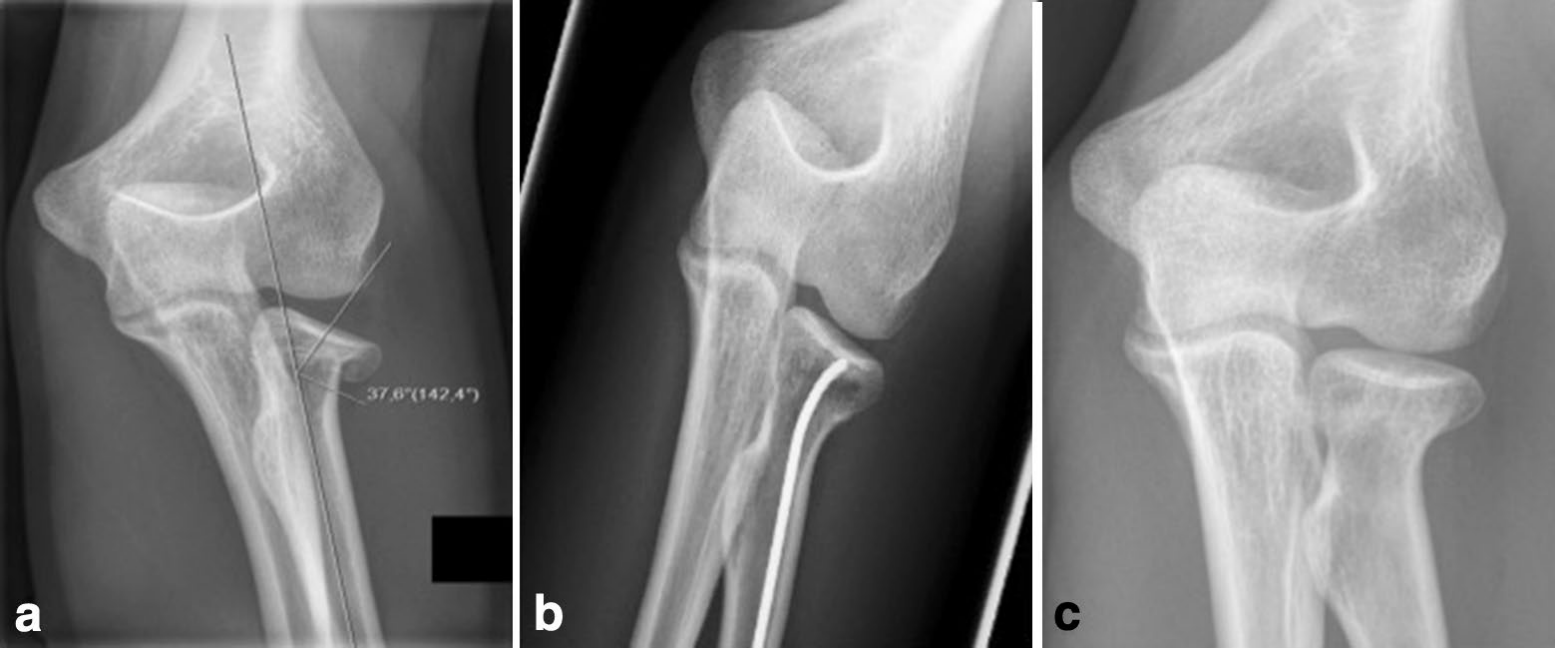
a.p. X-rays of patient nr 7 (see Table 1). This figure shows the a.p. X-rays of patient number 7. The patient suffered from a displaced Mason type III radial neck fracture (a). The postoperative results (b) following intramedullary pinning show a very good alignment. After implant removal (c) bony healing could be obtained, without a loss of alignment
